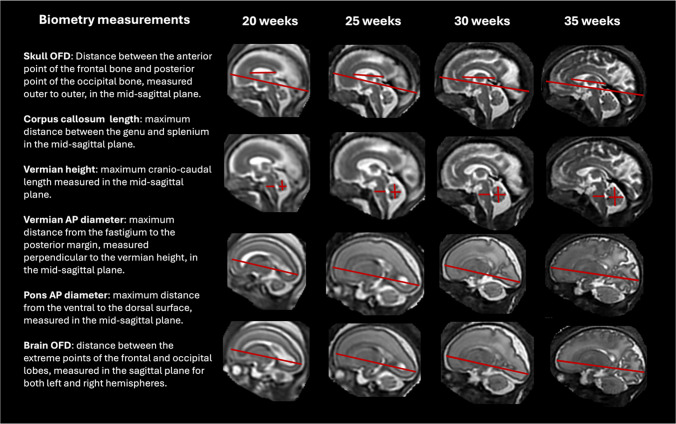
Proposed formalized protocol for automated brain biometry for 3-D T2W fetal MRI from 20 to 35 weeks GA range (sagittal planes, part 2). *AP* antero-posterior, *OFD* occipito-frontal diameter
